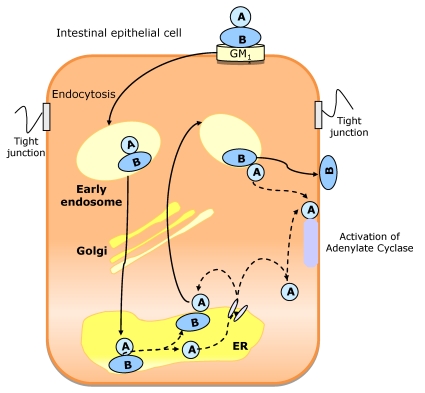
Proposed mechanism of cholera toxin (CT) traffic into intestinal epithelial cells. The CT holotoxin binds to GM_1_ in the apical membrane. After endocytosis, the CT-GM_1_ complex traffics retrograde through Golgi cisterna into endoplasmic reticulum (ER). Here, the A_1 _subunit is unfolded and dissociated from the B pentamer. The unfolded A_1_ peptide is probably translocated to the cytosol and may then gain access to its substrate, the heterotrimeric GTPase Gsα on the cytoplasmic surface of the basolateral membrane, by diffusion through the cytosol (if the A1 peptide breaks away from the membrane after translocation) or by membrane traffic back out of the secretory pathway (if the A1 peptide remains membrane associated). The B subunit is not unfolded in the ER, remaining membrane associated, probably bound to GM_1_. It moves to the basolateral membrane by trafficking back out the secretory pathway by indirect transcytosis.
